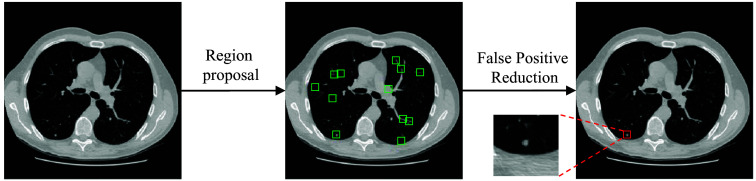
Overview of our lung nodule detection framework. The initial stage involves region proposal, which parses the volumetric CT scan with high sensitivity to identify potential nodule locations. Subsequently, the second stage aims for high precision, focusing on eliminating FPs generated in the first stage by classifying the ROIs defined by the initial stage detections as nodule or non-nodule regions.
